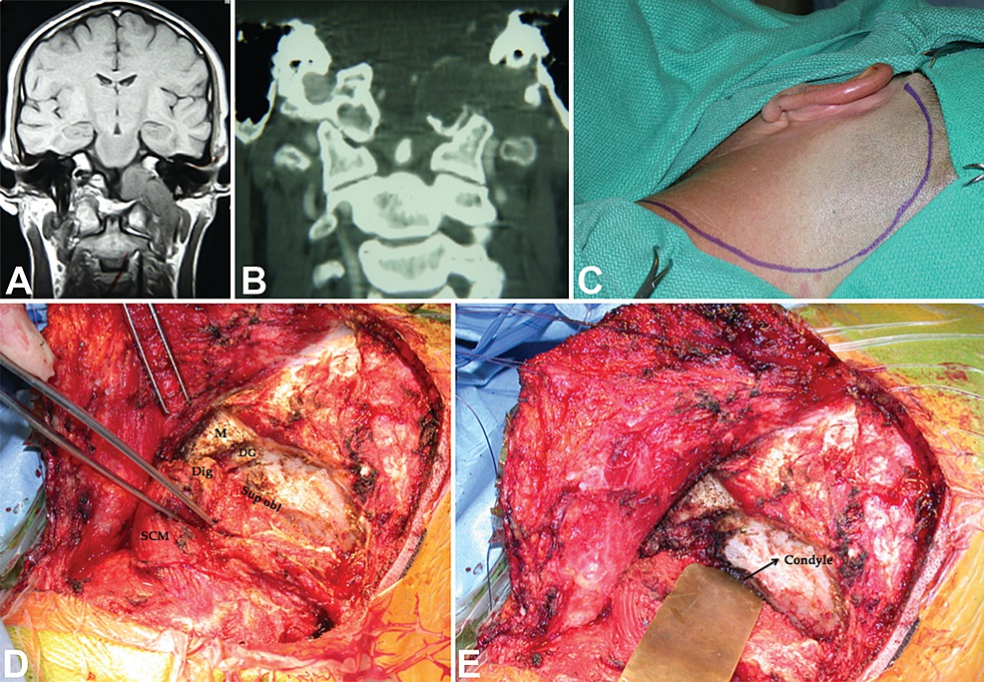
Hypoglossal schwannoma accessed via condylar triangle. Coronal T1 MRI (A) and coronal CT (B) scans showing a left hypoglossal schwannoma involving the cerbello-medullary cistern, occipital condyle, and hypoglossal canal, medial jugular foramen, and the distal cervical region. The 37-year-old man presented with headache, left neck pain, and hemiatrophy of the left tongue (case 32). A modified approach that combined a far lateral exposure was done (C, D, E) to identify the condylar triangle under the superior oblique muscle (sup obl). The intracranial, intraforaminal, and cervical components of the tumor were then completely resected. Labels: DG: digastric groove; Dig: digastric muscle; M: mastoid; SCM: sternocleidomastoid, Sup obl: superior oblique muscle.
